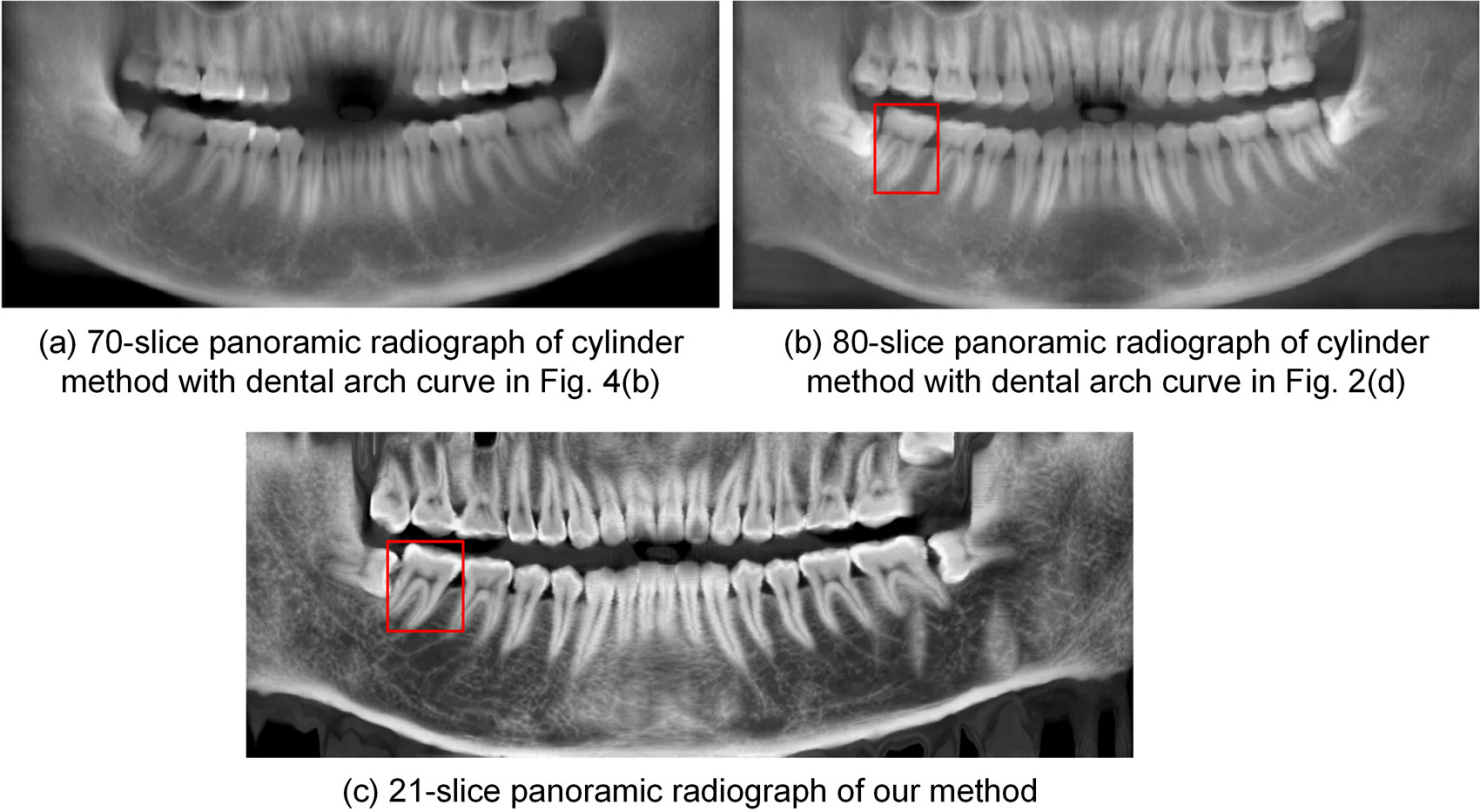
Thickened panoramic radiographs by different methods from dataset 1.
